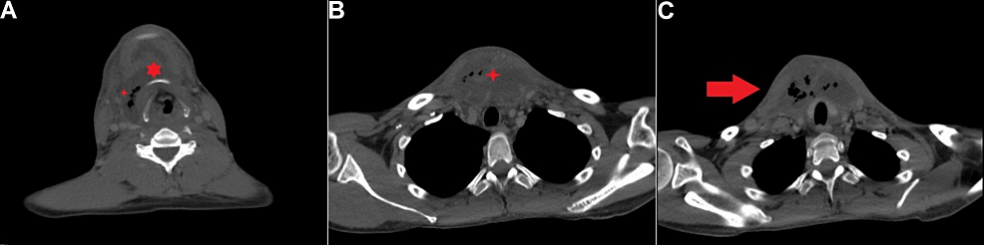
Neck and chest computed tomography (CT) scan A: Neck CT scan at the level of C4, showing a bilateral submandibular and parapharyngeal neck abscess, more on the right side, with gas foci causing obliteration of the right parapharyngeal pad and deviation of the larynx to the left side.
